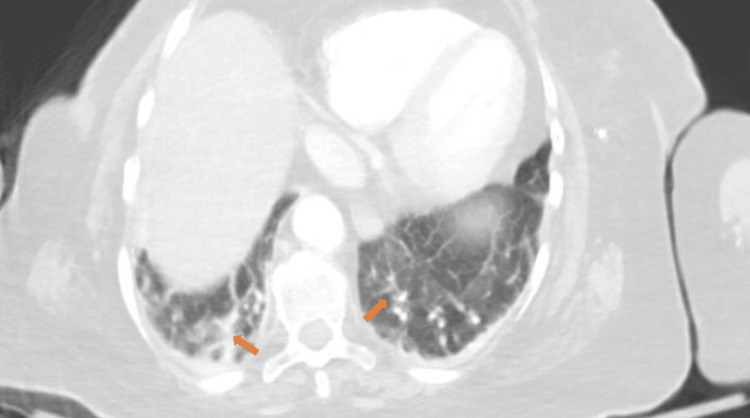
Bilateral pulmonary scarring and atelectasis on computed tomography (CT) scan (arrows).
